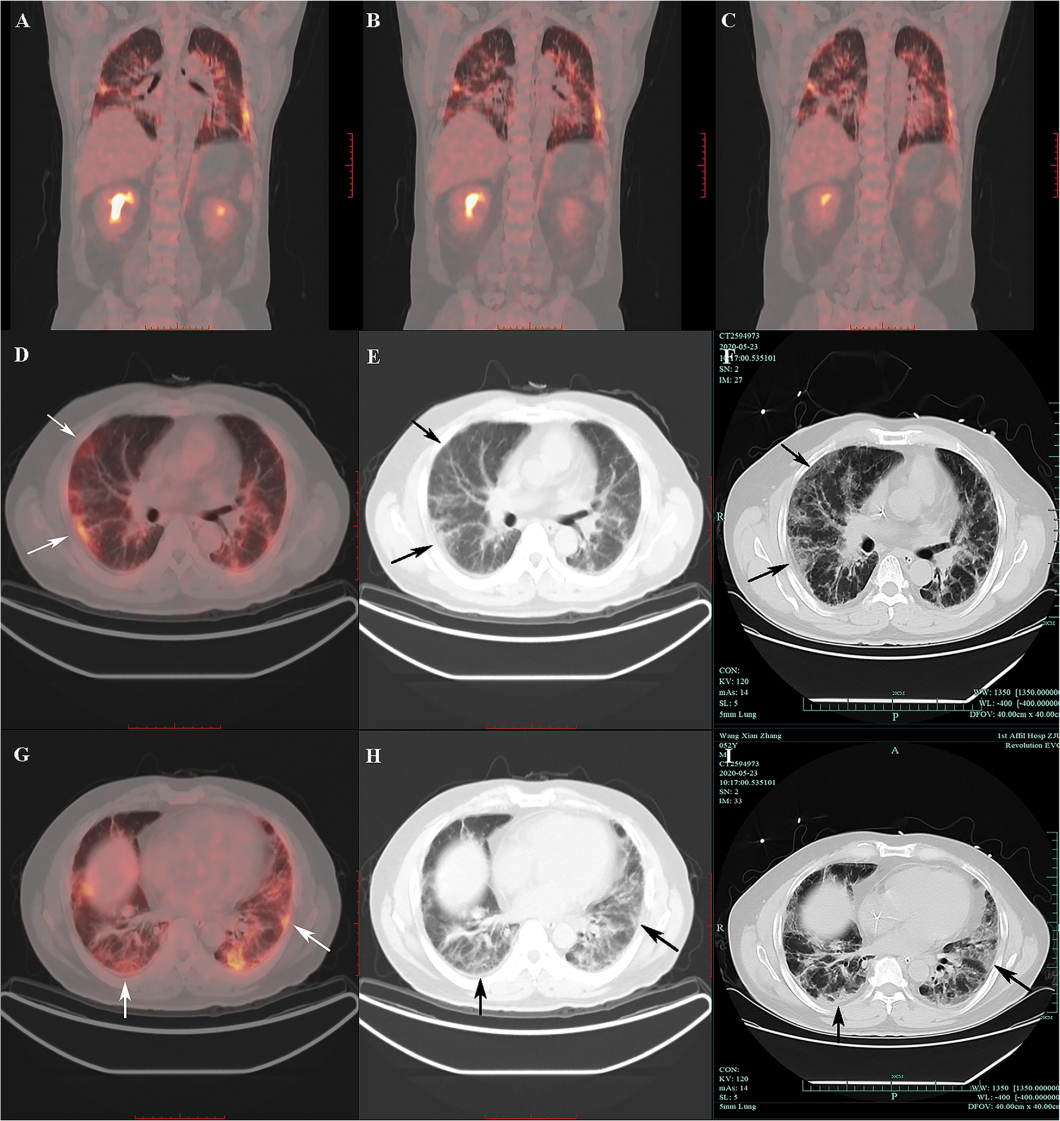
Visual examination of ^18^F-FDG-PET/CT scan and HRCT of one IIM-ILD patients who developed RP-ILD. A to C. Prominently elevated FDG uptake in bilateral lungs of one IIM-ILD patient who later developed RP-ILD. D to F and G to I. Prominently elevated FDG uptake in bilateral lungs of one IIM-ILD patient indicated future RP-ILD in one week (where the arrow pointed). FDG: Fluorodeoxyglucose; HRCT: High resolution CT; IIM-ILD: Idiopathic-inflammatory-myopathy-related interstitial lung disease; RP-ILD: Rapidly progressive interstitial lung disease
